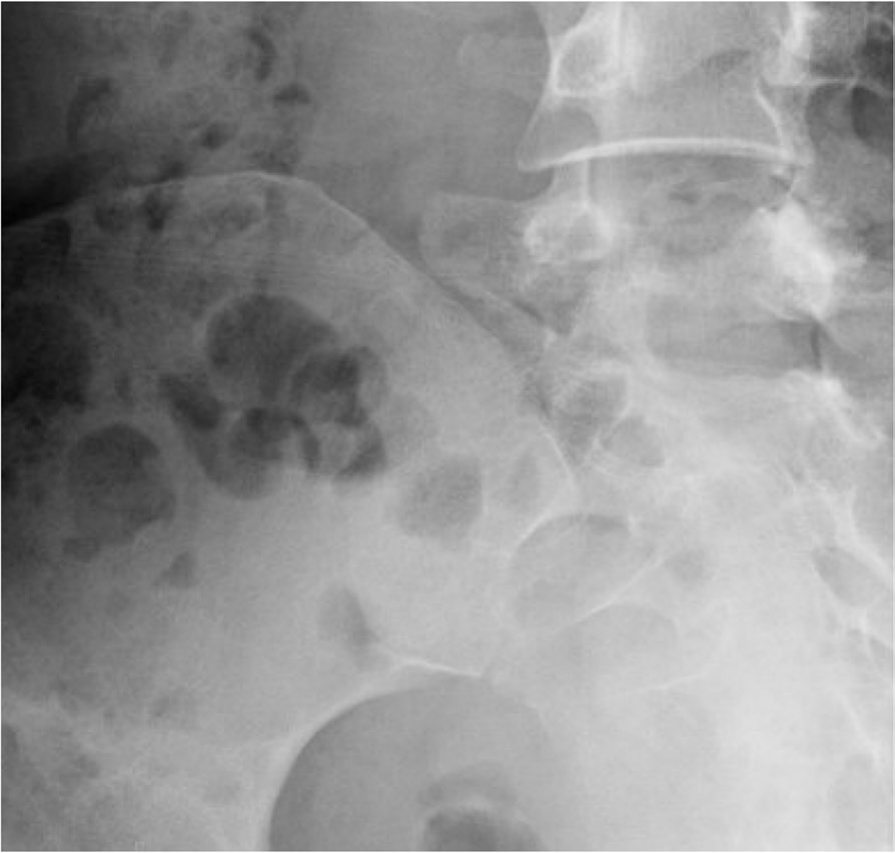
Spondylolisthesis. Anterior translocation of L5 vertebrae is appreciable on false-profile hip radiographs
